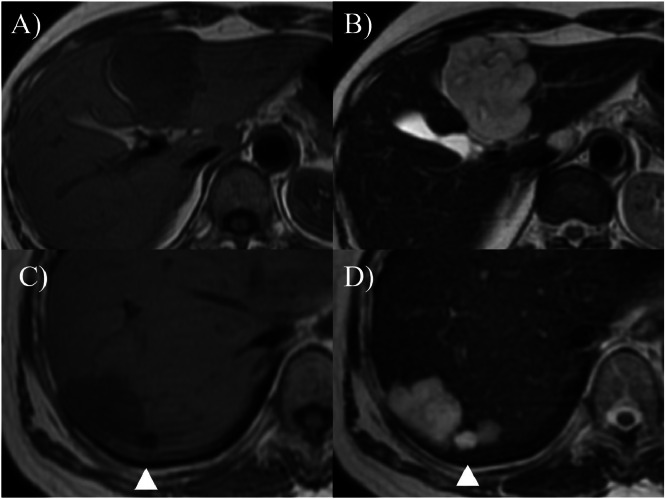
Dynamic abdominal magnetic resonance imaging findings (2007+6 years). A mass of 55×43 mm is observed in liver S2/3 (A, B) and 36×30 mm in liver S8 (C, D). T1WI shows low signal intensity (A, C), and T2WI shows high signal intensity (B, D). A 9 mm cyst is observed near S8 (arrowhead).
